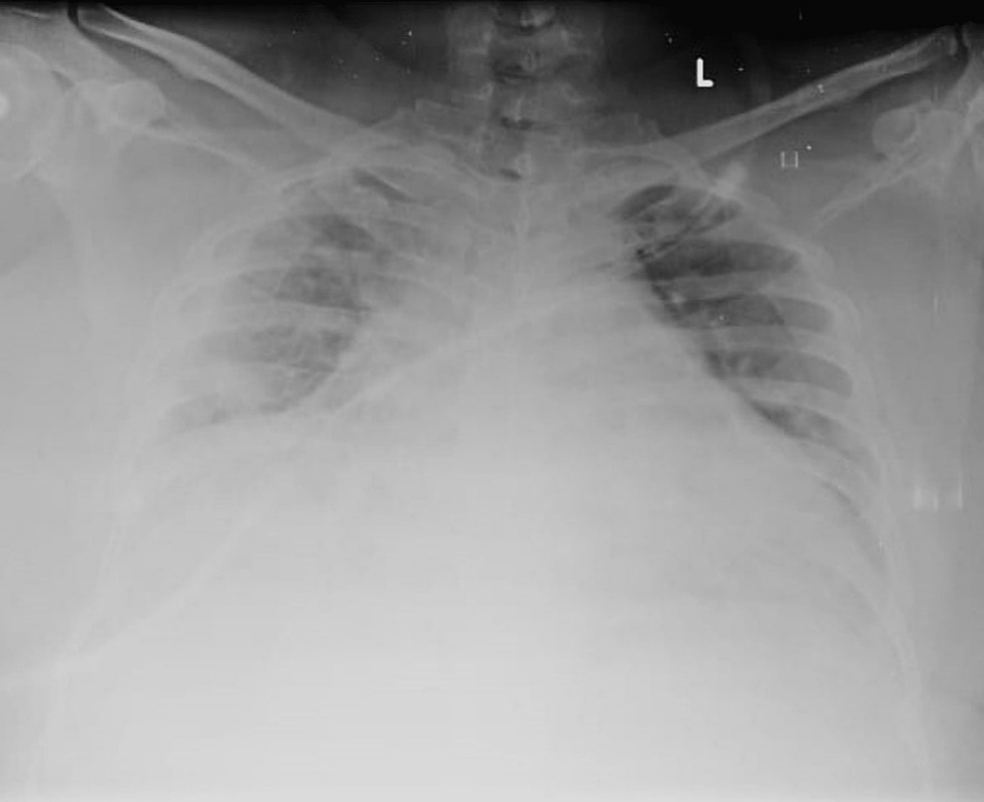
Chest X-ray at discharge: re-expansion of the right lung field.
